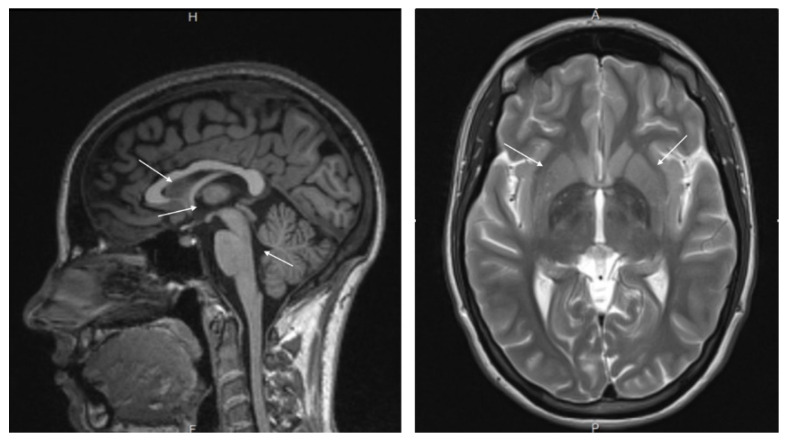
MRI showing atrophy, and functional asymmetry.
